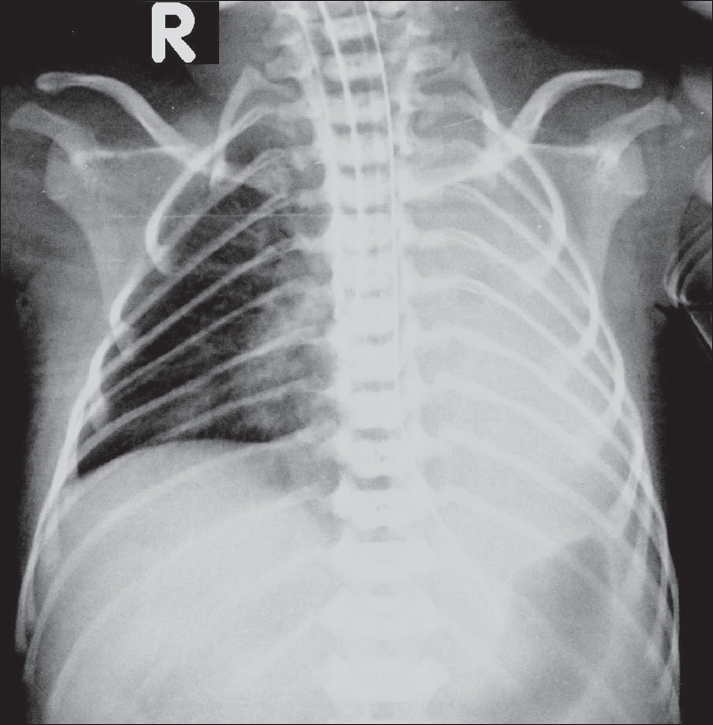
Chest radiograph on day 2. Note the left lung collapse
